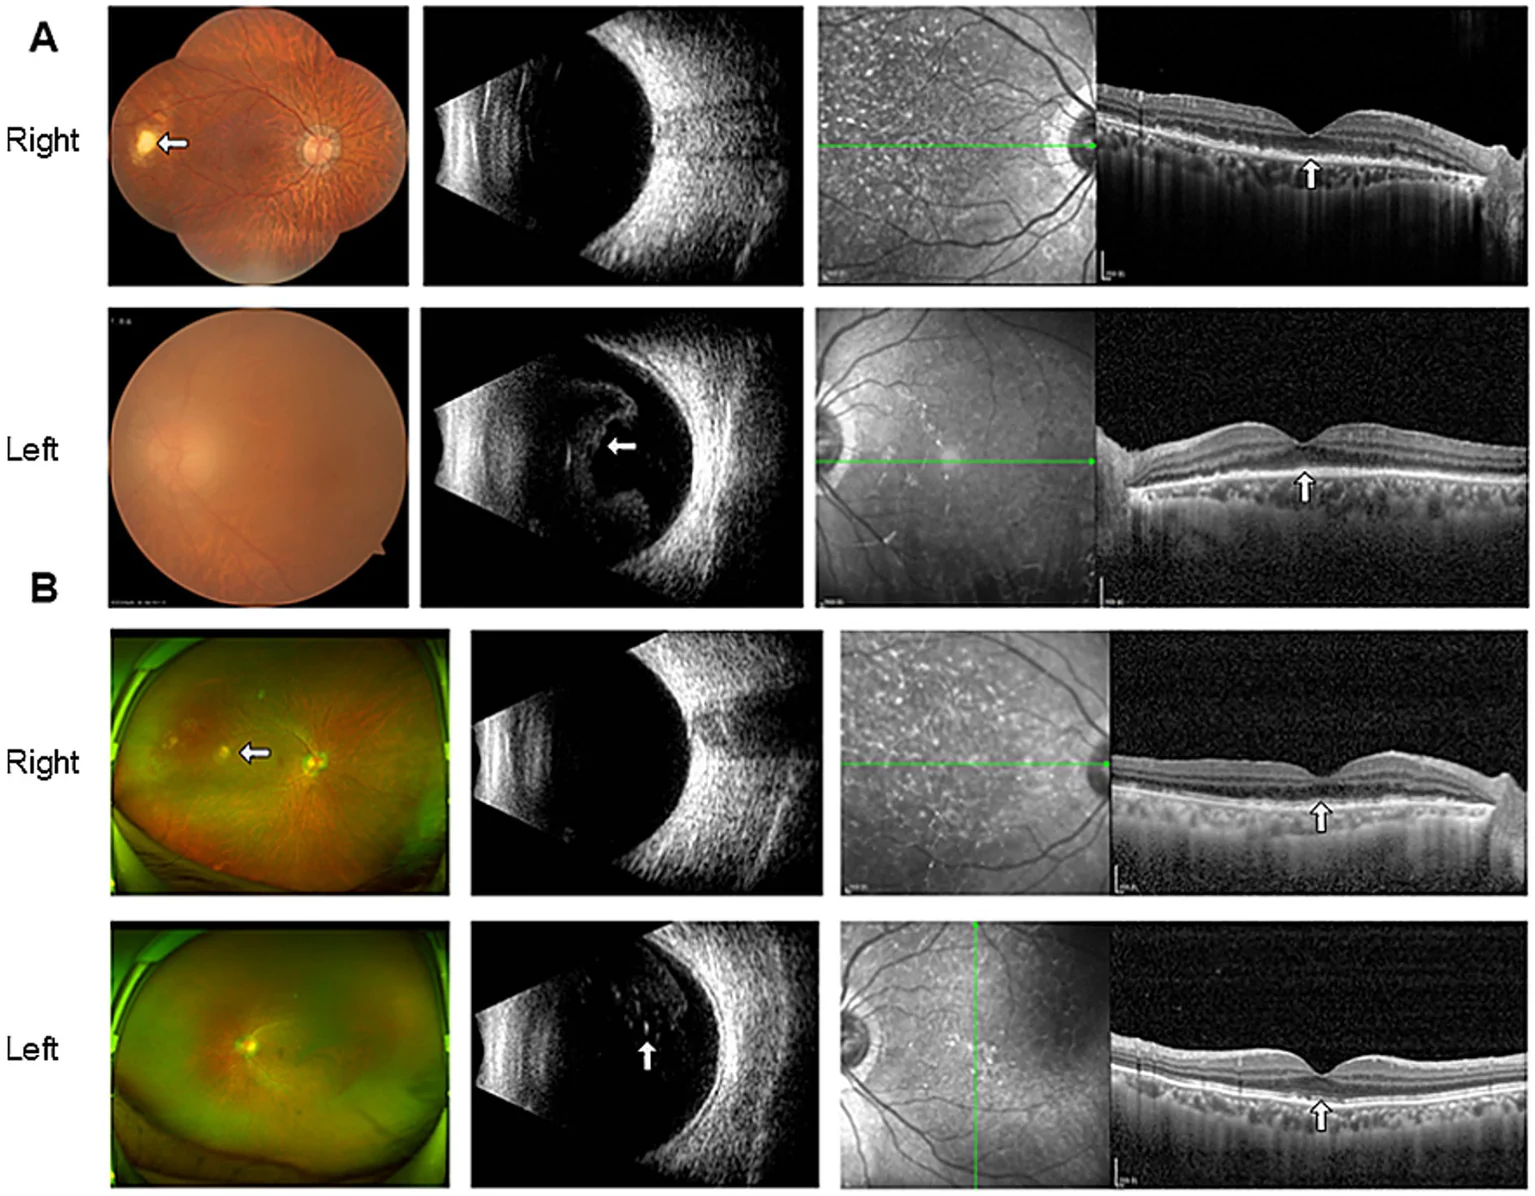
Positron emission tomography-computed tomography (PET/CT) results. The density distribution of the vitreous humor in both eyes is uneven, with a slight increase in FDG uptake, and SUVmax/av. = 2.4/1.8. No abnormal FDG uptake was observed in other systemic organs, confirming that the disease was confined to the ocular compartments. (A) Results from admission on September 9th, 2022. (B) Results after the eleventh injection on October 14, 2022.
